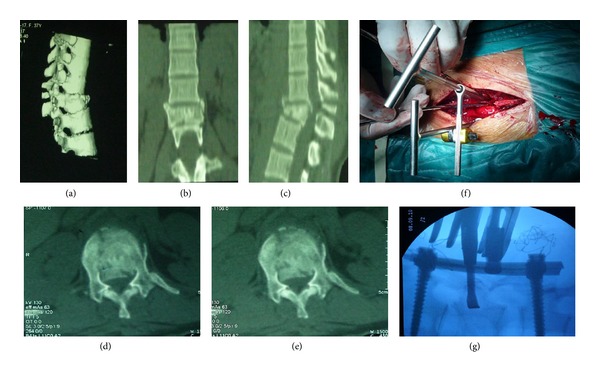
(a)–(e) The preoperative CT scan image demonstrating a significant spinal canal encroachment by retropulsed bone fragments. (f)-(g) The intraoperative application of RRBF and the fluoroscopy of restoration of fractured vertebral body.
